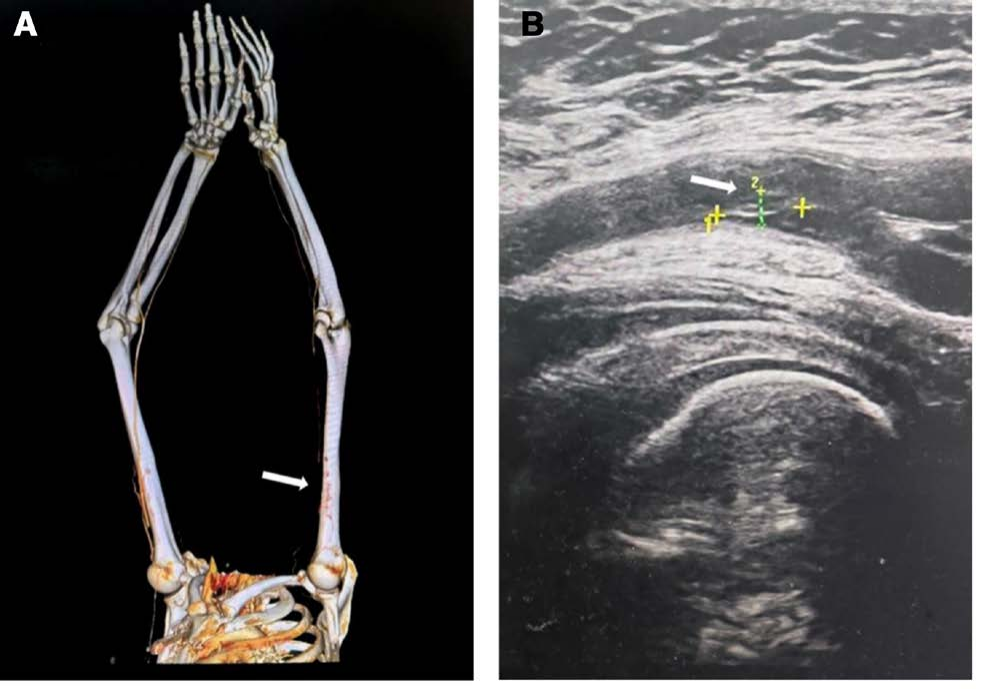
Results of medical imaging examination. (A) Vascular CTA of the upper extremity. There is a long-segment occlusion of the left axillary artery and the origin of the brachial artery (white arrow). (B) Vascular Doppler ultrasound of the lower limbs. Hypoechoic plaque is detected in the left femoral artery (white arrow). CTA = computed tomography angiography.
